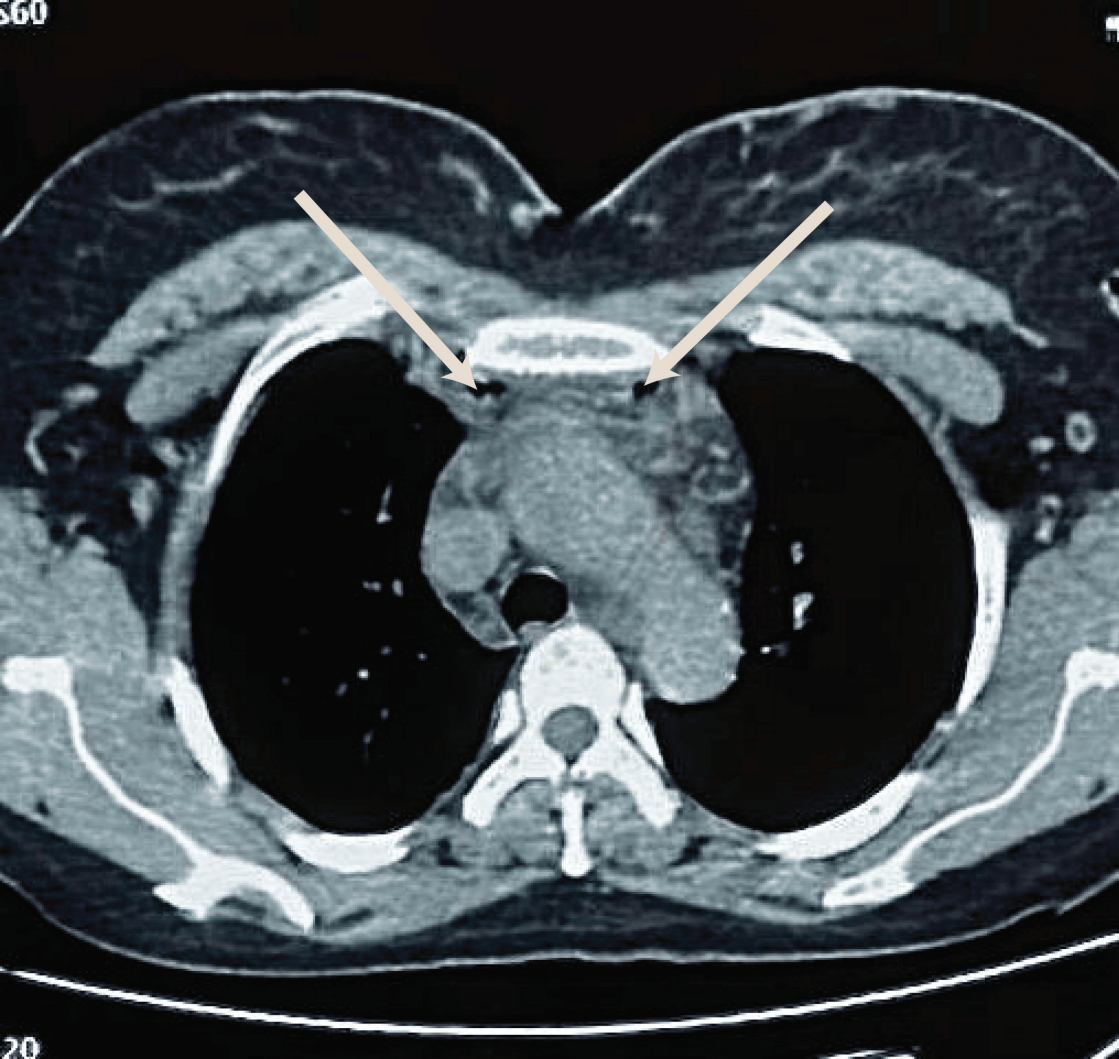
Axial thoracic CT demonstrating a hypodense collection with air bubbles in the anterior mediastinum
